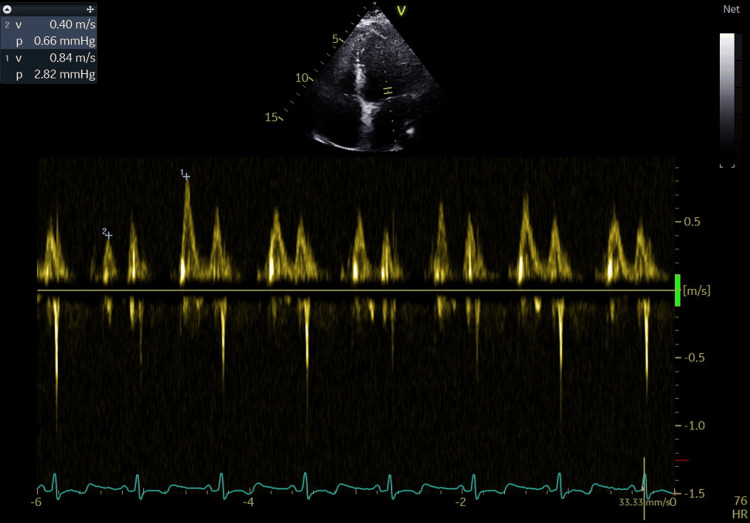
Pulsed Doppler mitral inflow shows 55% respiratory variation, exceeding the ≥25% threshold, suggestive of constrictive pericarditis. The variation reflects enhanced ventricular interdependence, with decreased left ventricular filling during inspiration and increased filling during expiration due to the non-compliant pericardium
